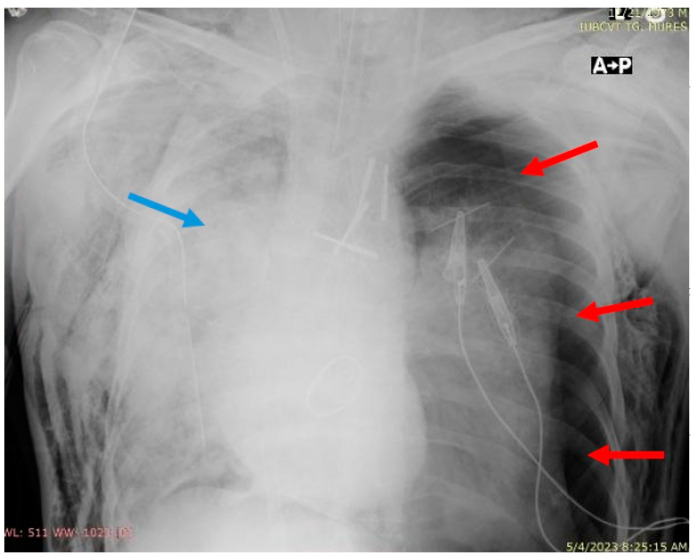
Thoracic X-ray revealing left pneumothorax (red arrows), UPE (blue arrow).
